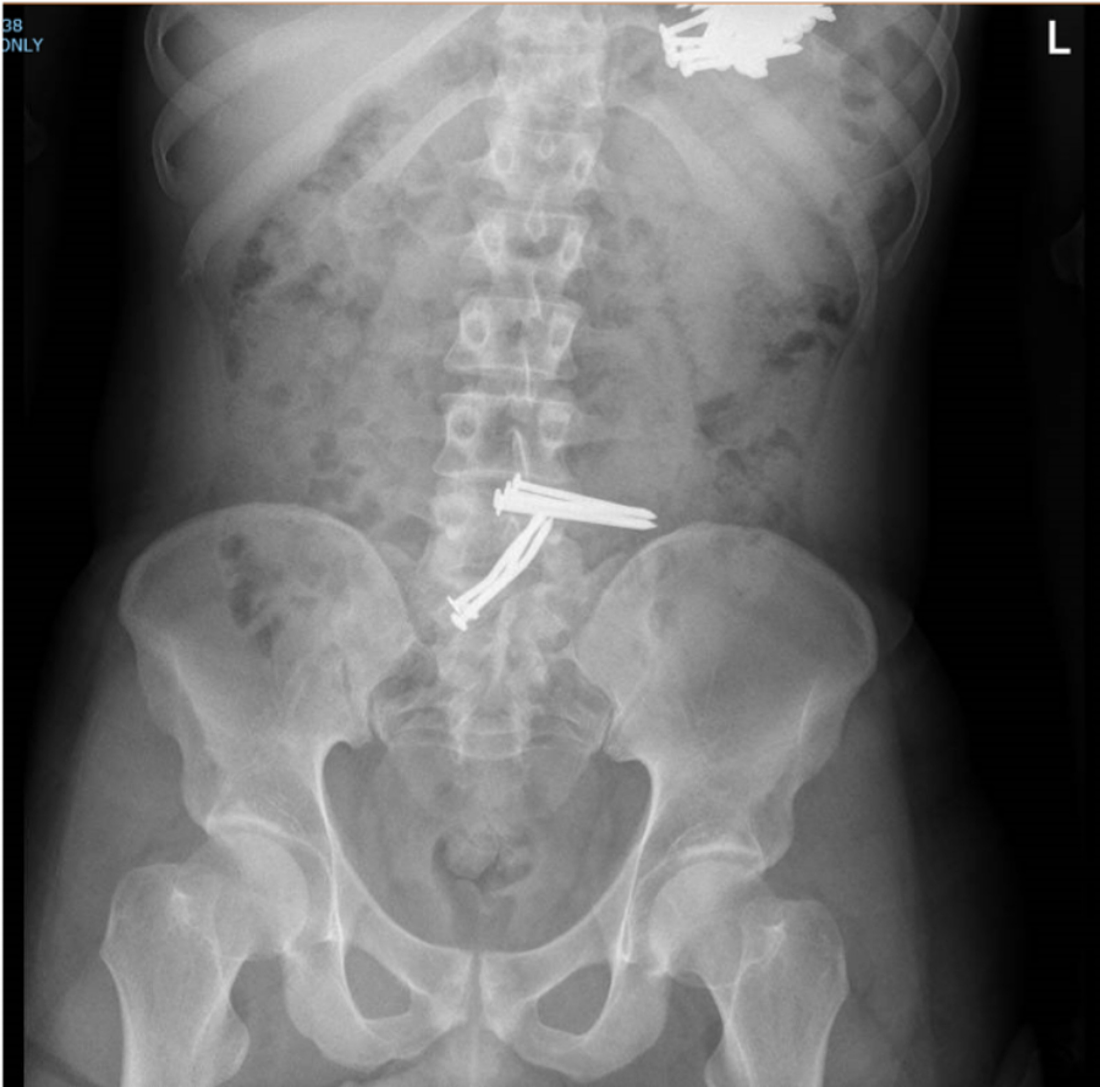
Plain abdominal X-ray showing multiple metallic nails within the stomach and the small bowel
